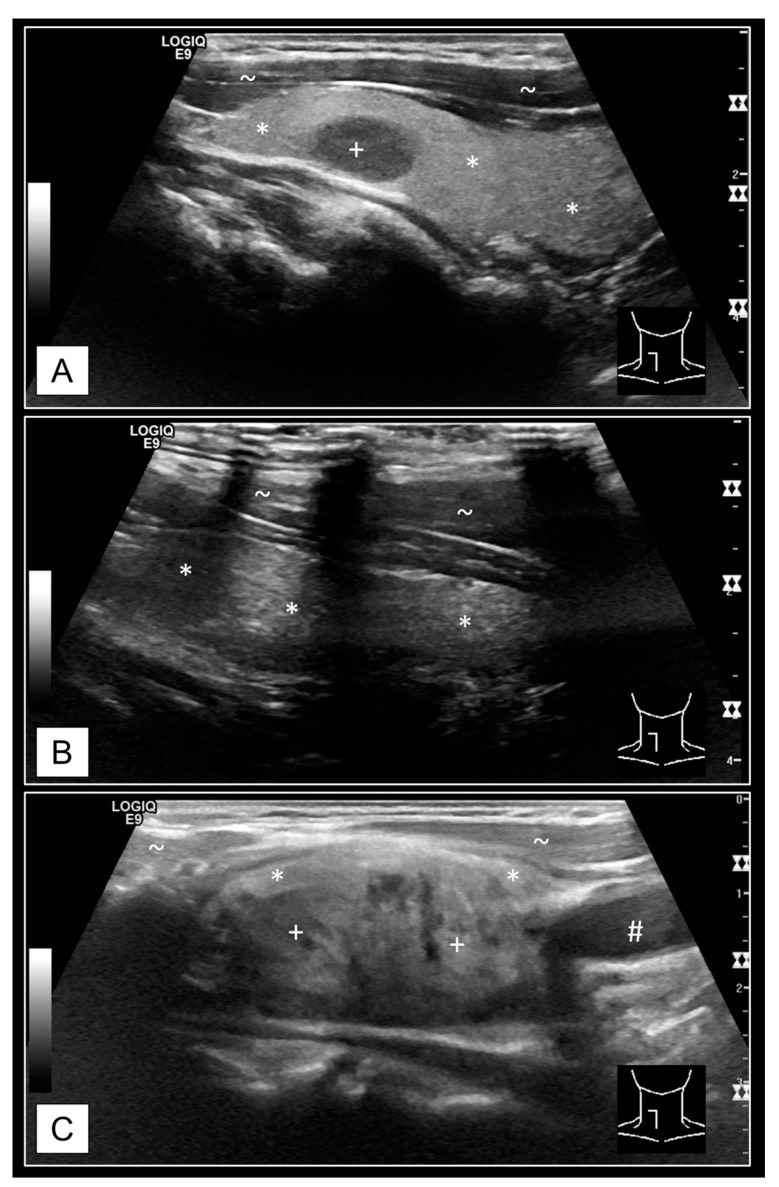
Examples for defiances on MTA US cine loops. (A) The caudal pole was missing; this is commonly due to breath-depending mobility of the thyroid gland. Patients should be asked to hold their breath during cine loop acquisition. (B) US artifacts caused by insufficient connectivity between the US probe and the patients’ skin via the applied ultrasonic gel. (C) Too fast movement of the US probe can lead to blurred images. This occurs especially with multiple depth foci (*n* = 3 in this case). Separate US pulses are generated for every focus, influencing the image frame rate. Thus, thyroid nodule contours/ borders cannot be accurately assessed on single images. Labels: * thyroid tissue, + thyroid nodules (TN), # carotid artery, ~ muscle tissue.
